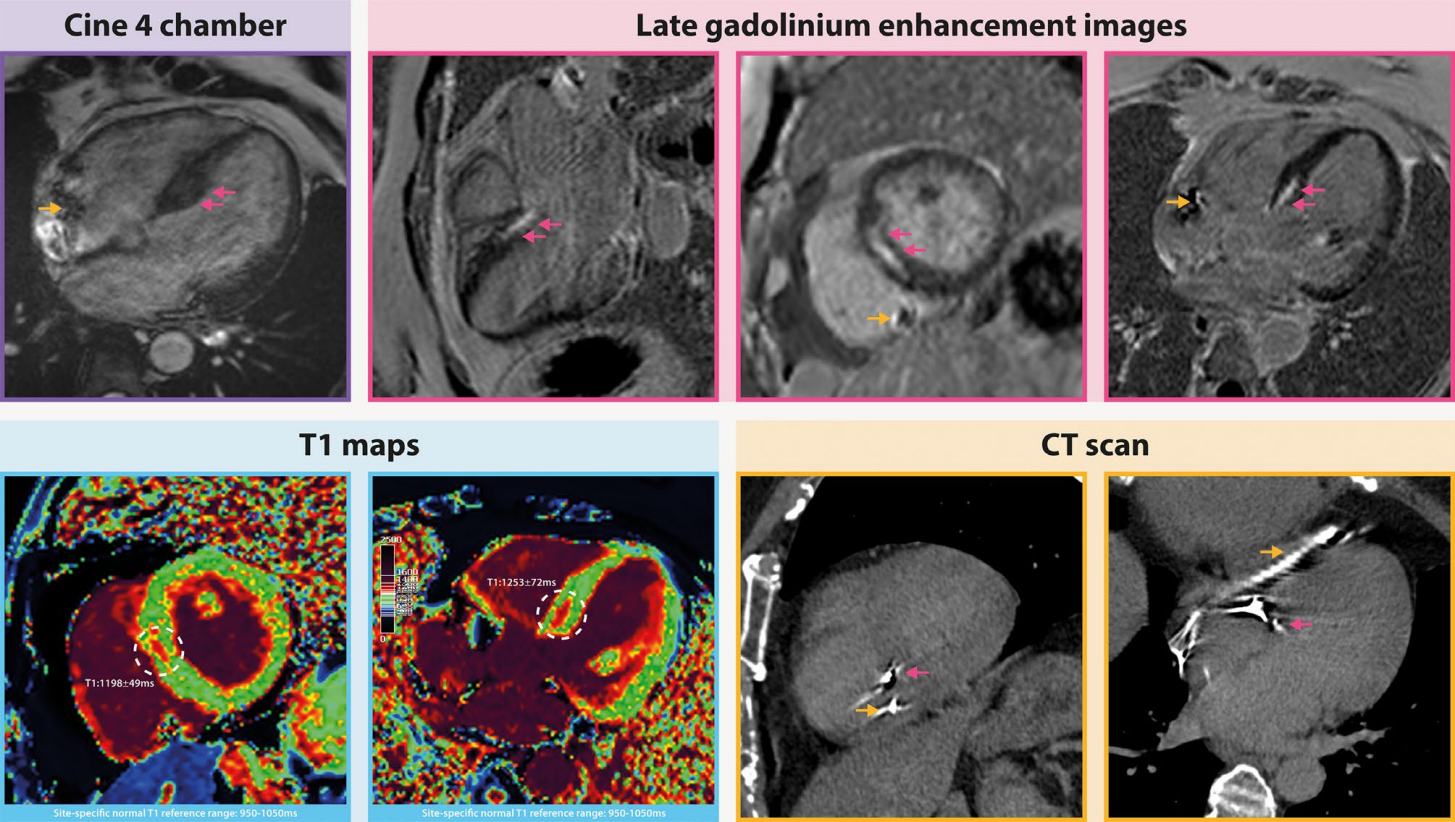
Cardiovascular MRI scan. The 4-chamber cine image showed proximal septal thickening (purple arrows) and the artifacts of the leads (yellow arrows). Late gadolinium enhancement images displayed a patchy non-ischemic mid-myocardial high signal intensity in the same proximal septum (purple arrows). T1 mapping demonstrated focal high T1 values in the proximal septum of 1263 ± 51ms (site-specific normal myocardial T1 values: 950–1050ms). On the CT scan, the lead artifacts are distinctly discernible (purple arrow is the LBBA lead, yellow arrow is the RV lead), providing a clear indication of the point at which the lead was inserted into the right ventricular septum. The insertion site aligns with the corresponding late gadolinium enhancement images within the same image plane. Notably, the late gadolinium enhancement exhibits a broader extent than the lead insertion site, encompassing both the infero-antero septal and basal-to-mid septal directions
